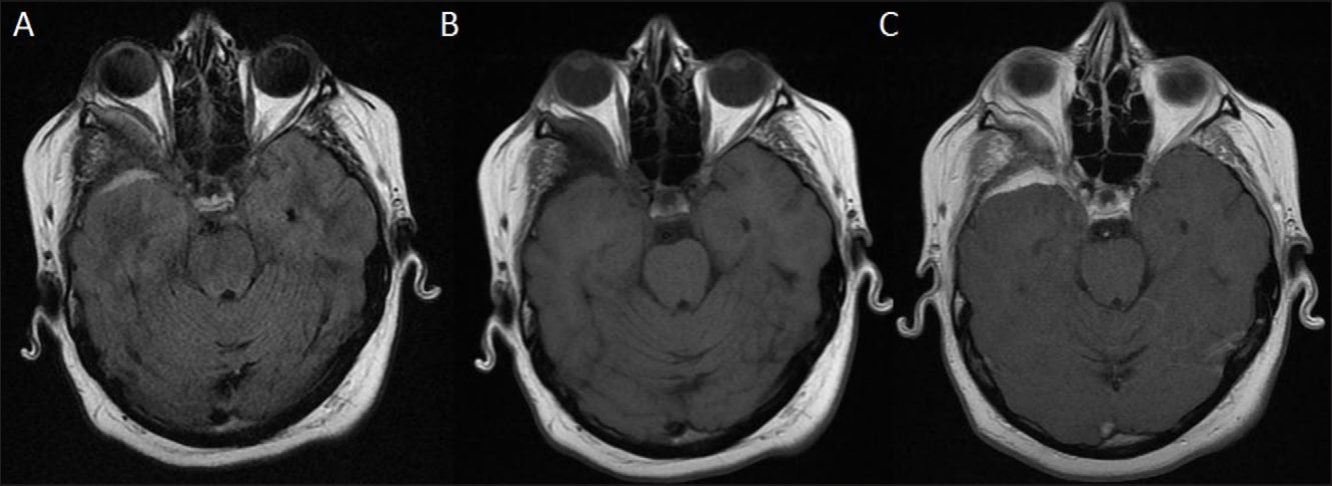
MRI of the brain with axial FLAIR (A) and unenhanced and contrast-enhanced axial T1-weighted images (B, C) shows right-sided anterotemporal FLAIR-hyperintense contrast-enhancing dural thickening abutting the bony sphenoid changes.
